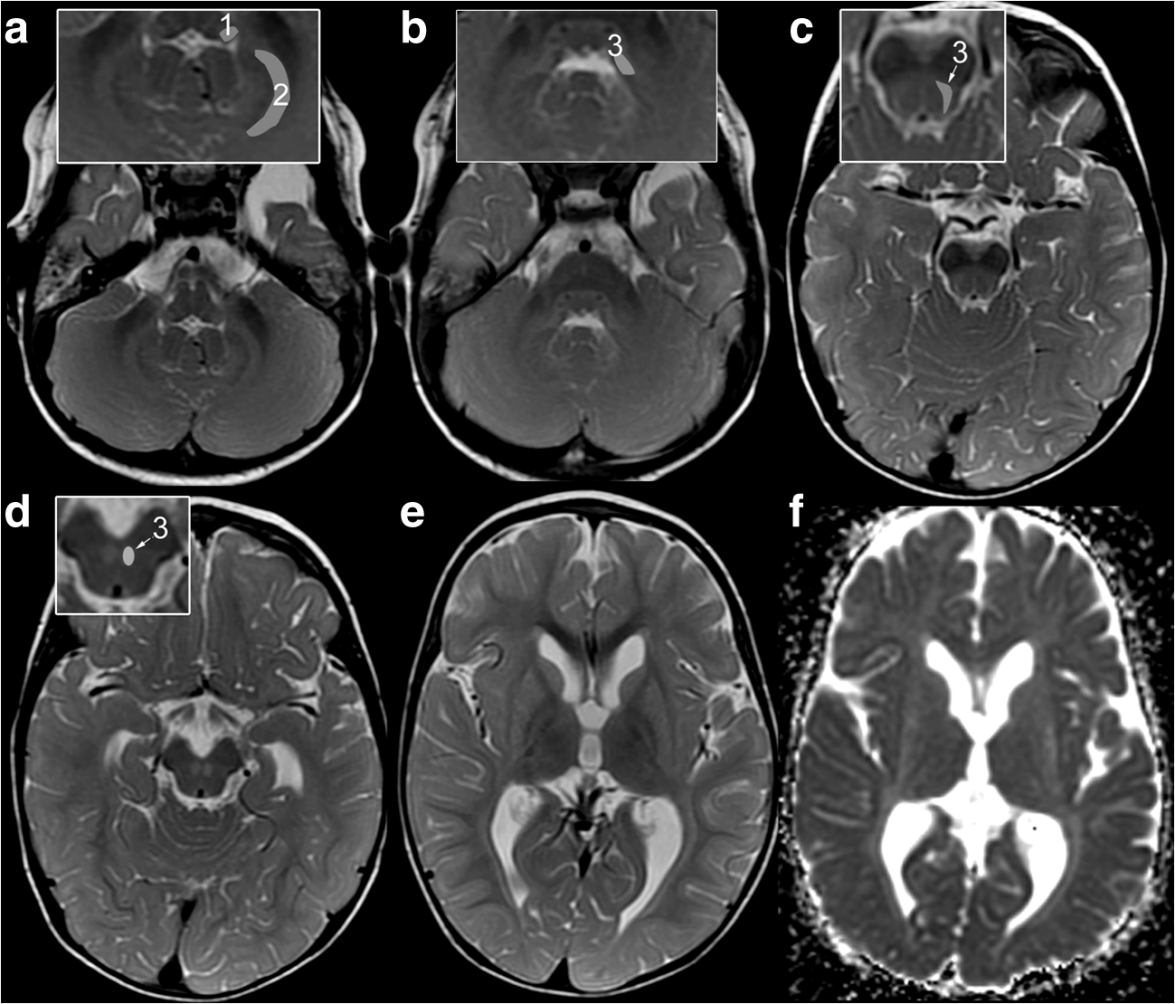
Characteristic MRI pattern of striatal variant of POLR3A-related disease. MRI in patient 1 at 1.5 years demonstrates the characteristic combination of atrophic, T2-hyperintense striatum ,and T2-hyperintense SCP (A-E: T2w; F: ADC-map; insets: 1 = ICP, 2 = peridentate white matter, 3 = SCP). a T2-hyperintensity of ICP (1) and peridentate white matter (2) are additional findings. b Further additional findings are T2-hyperintensity of tegmentum and intraparenchymal course of trigeminal nerve. T2-hyperintensity of SCP (3, insets in B-D) is seen along its course from the cerebellum (b), dorsal mesencephalon (c) to the decussation in the anterior mesencephalon (d). e, f: Homogeneous, mild, and symmetric T2-hyperintensity of the striatum with volume loss and increased diffusion. NB the lateral medullary lamina between pallidum and putamen is commonly seen at this age due to its relative T2-hyperintensity compared with pallidum and putamen; increased conspicuity is due to T2-hyperintensity of putamen (e)
